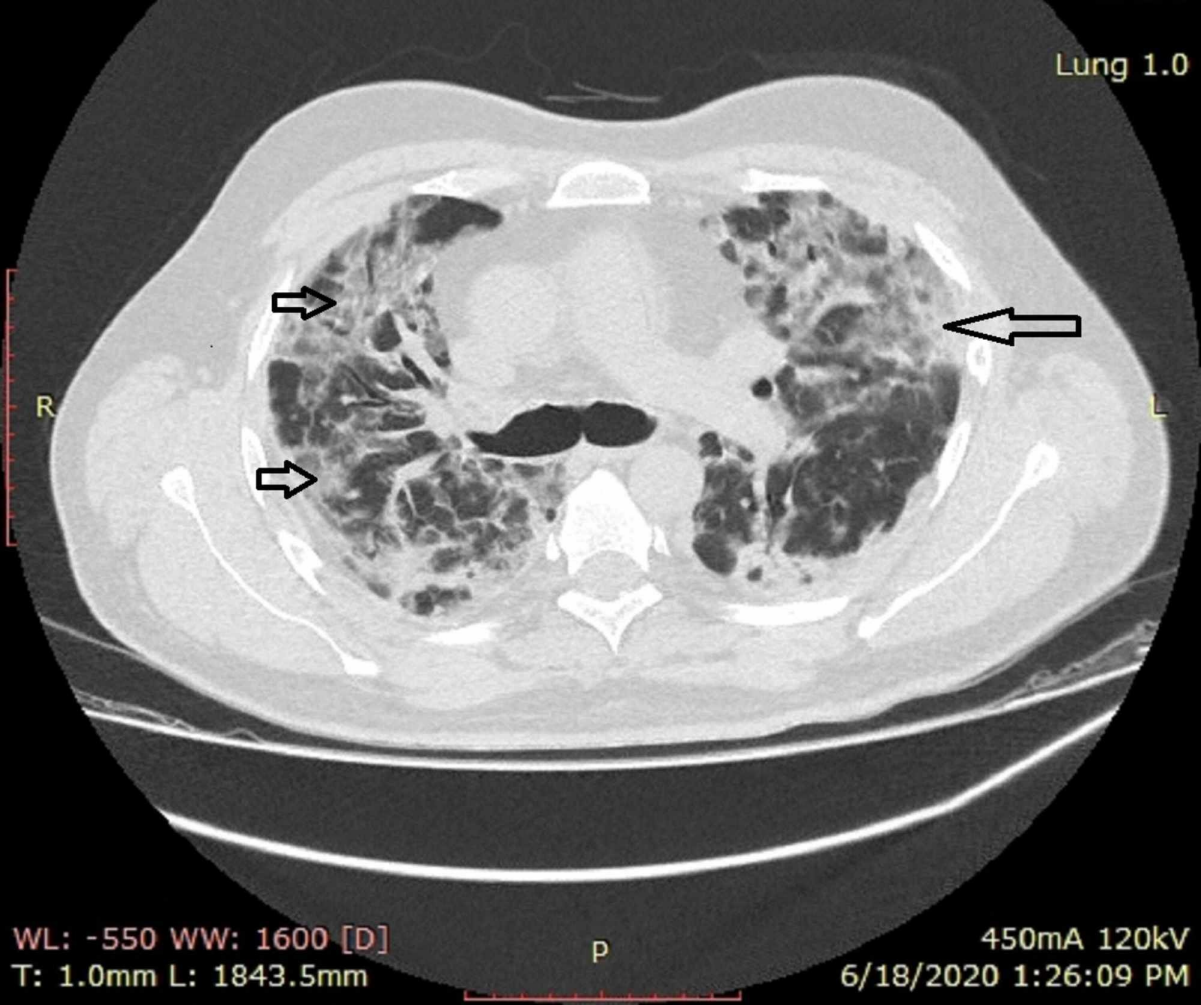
A male patient with severe disease HRCT shows multiple bilateral areas of ground-glass opacities and consolidation in bilateral lungs (short and long arrows) HRCT: high-resolution computed tomography
